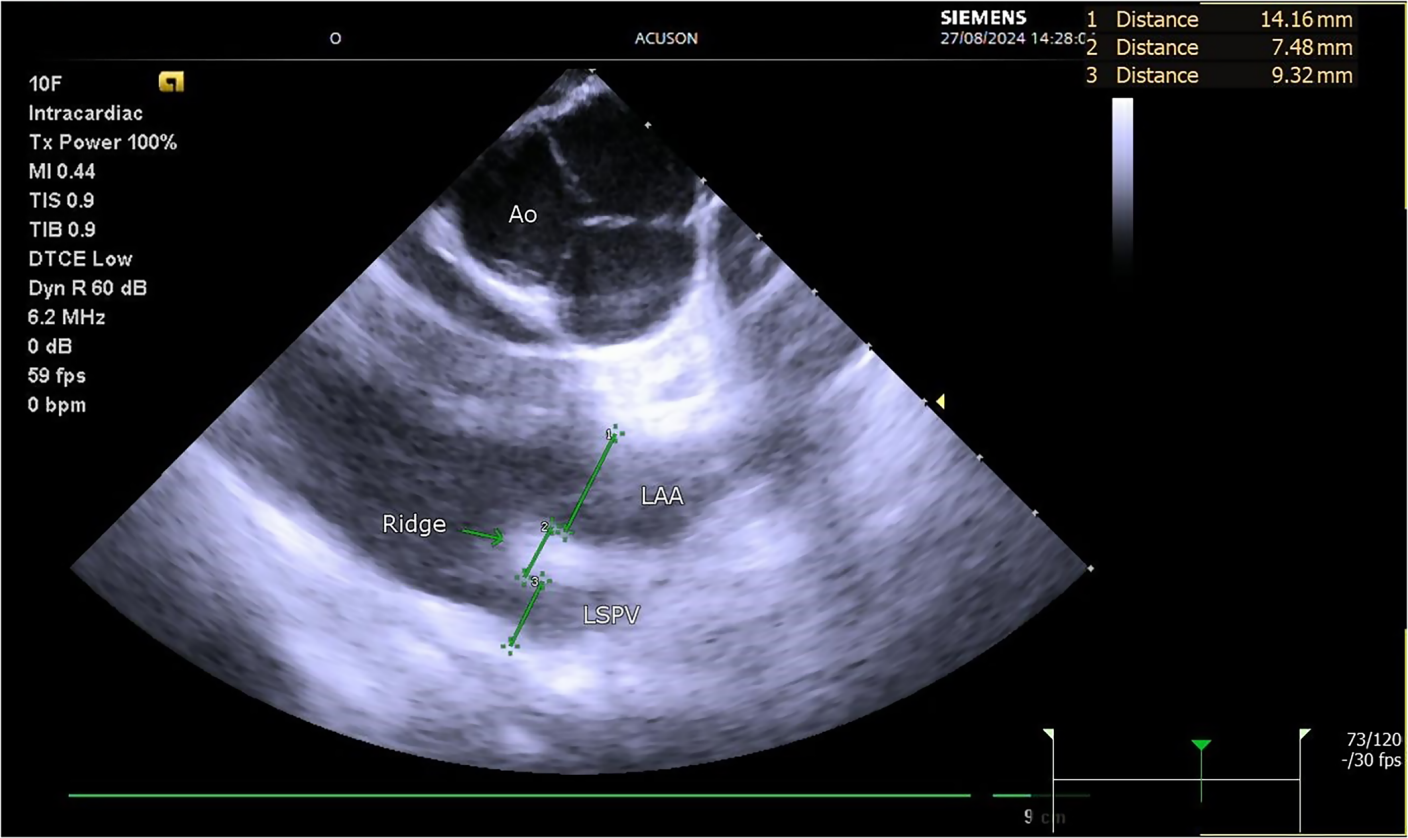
Intracardiac echocardiography measurements from the RVOT. Abbreviations: Ao, aorta; LAA, left atrial appendage; LSPV, left superior pulmonary vein.
